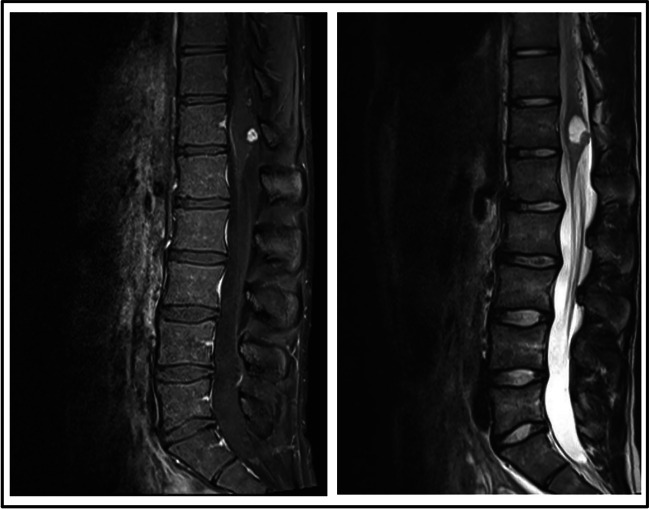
Contrast-enhanced T1 and T2 MRI sagittal images obtained from patient III-1, showing a hemangioblastoma with associated cyst located in the conus medullaris that required surgery
